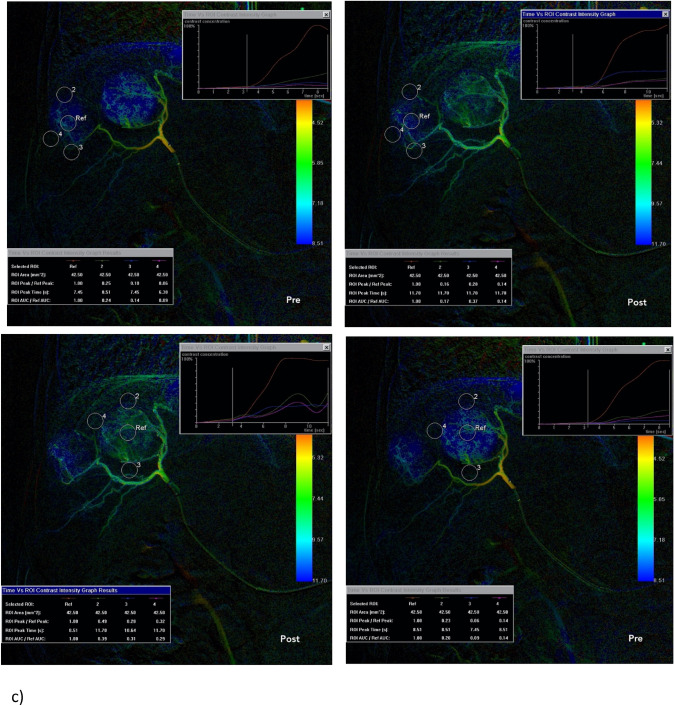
a CT scans showing three hypervascular HCC nodules located in segment 8 and 7 as confirmed by selective angiography performed in the common hepatic artery. b Superselective catheterization performed with balloon microcatheter Occlusafe^®^ (Terumo, Japan). The angiography performed with inflated balloon (post) detects more feeding vessels compared to angiography performed with deflated balloon (pre). c Syngo iFlow is applied to selective angiographies, performed before embolization with both deflated and inflated micro-balloon, showing an increase of peak time in the ROI-t located in the centre of tumour and the ROI-ihl located in the healthy parenchyma of the same segment
